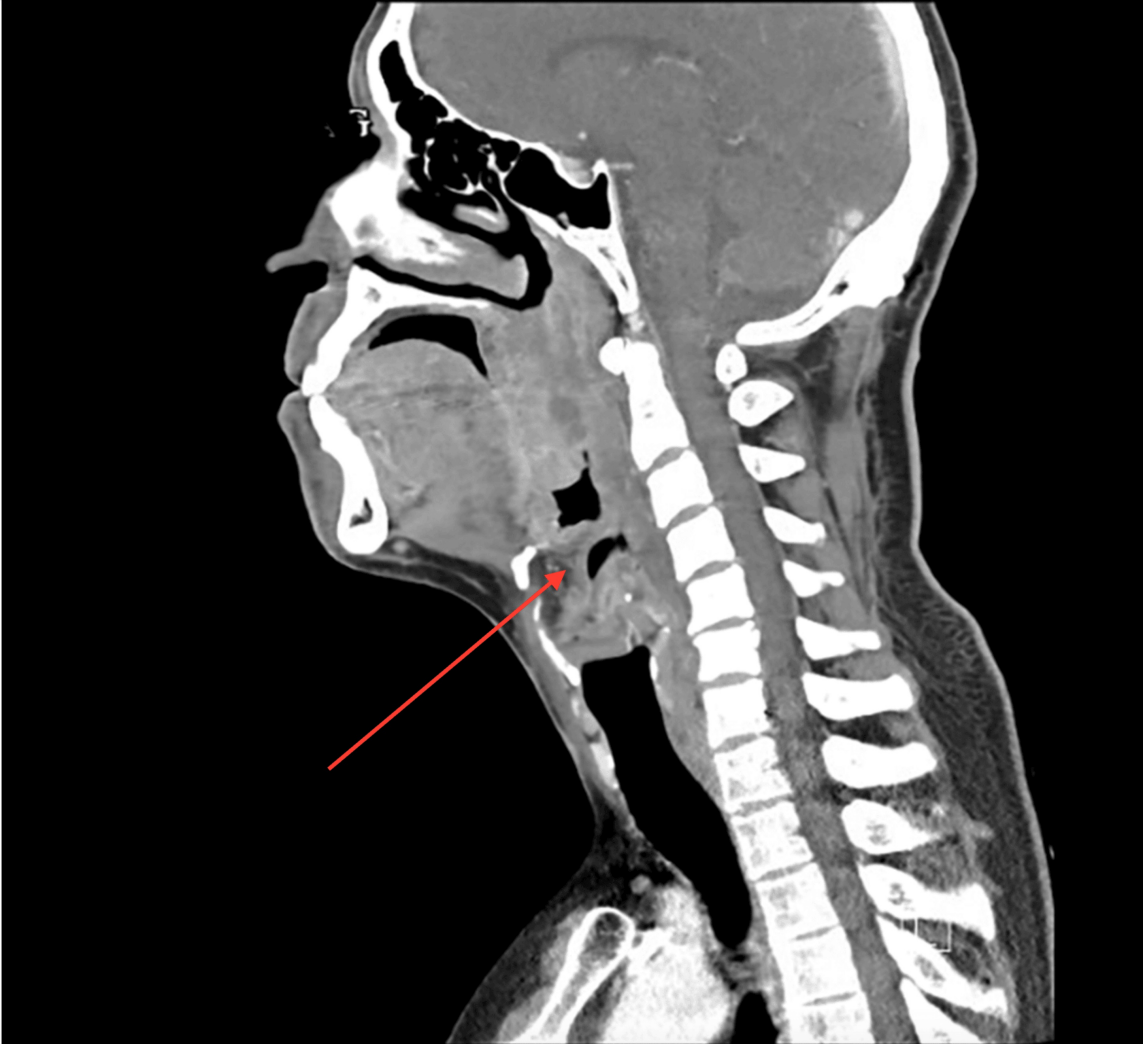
Contrast-enhanced sagittal CT of the neck demonstrating thickening of the epiglottis indicated by arrow.
